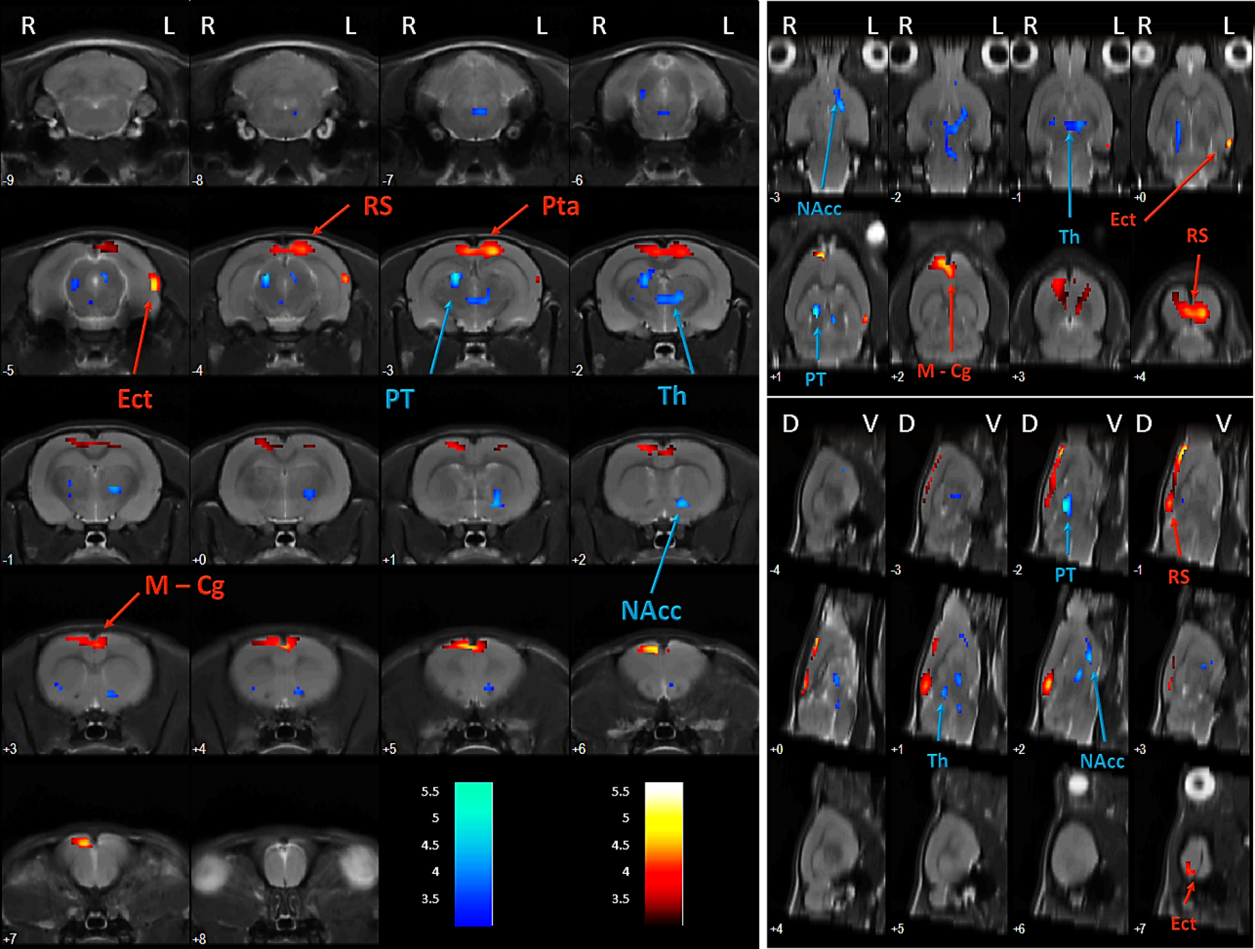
NAcc-DBS effects on brain metabolism. Axial (left), coronal (upper right) and sagittal (lower right) views of the brain. Colored PET overlays on the MR reference indicate increased FDG uptake (hot colors) or decreased FDG uptake (cold colors) 1 day after the end of the stimulation in NAcc. Left (L), right (R), dorsal (D), ventral (V).
